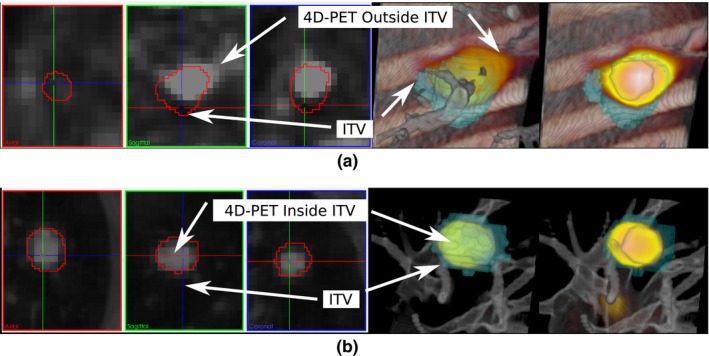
Example visualization 4D‐PET/CT and ITVs: 2D views and with 4D‐VS. Examples show a rejected ITV (a) with a DC = 0.65 and an accepted ITV (b) with a DC = 0.76.
